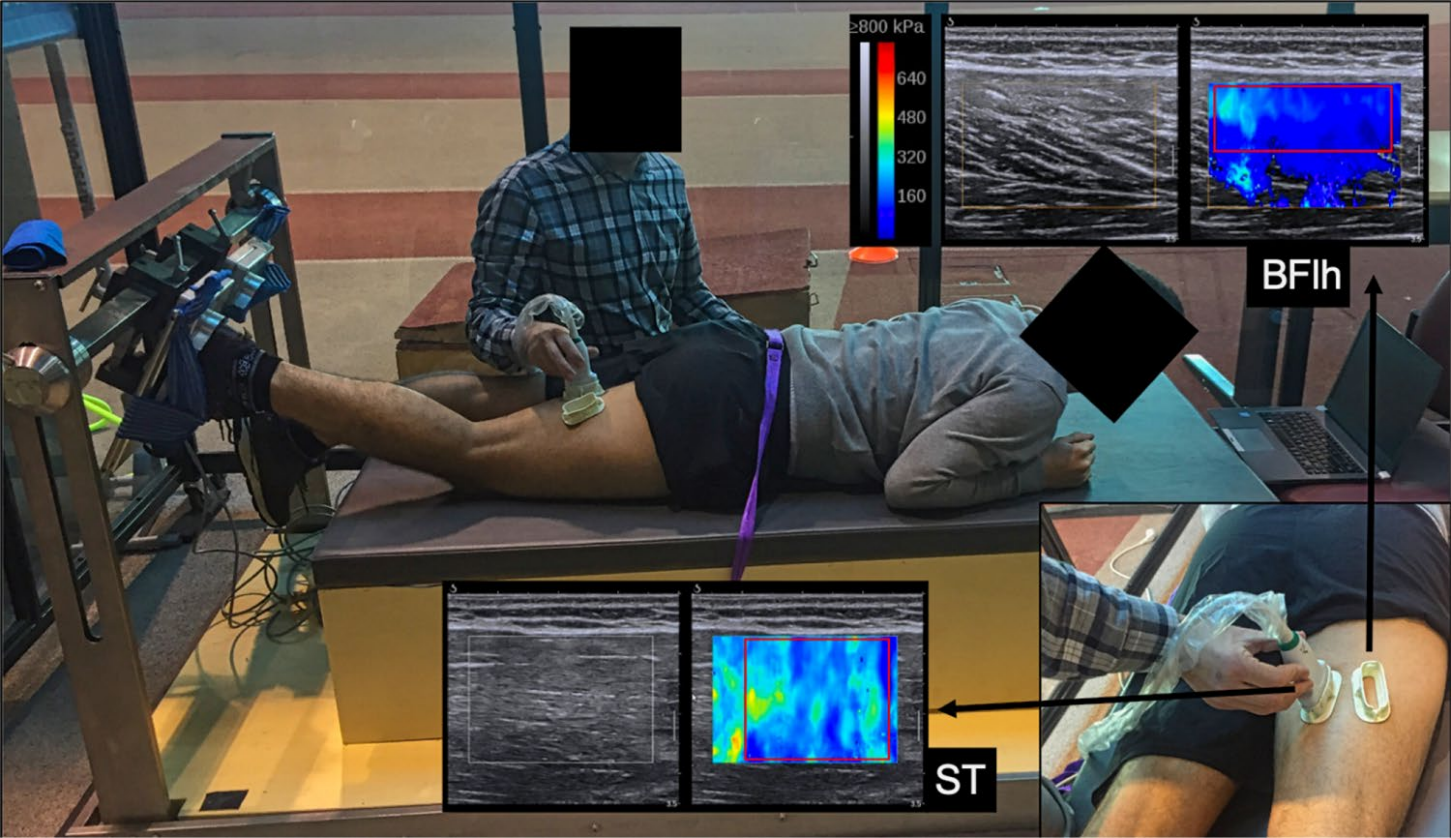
Experimental setup used to assess the localized active stiffness (i.e. shear modulus) of biceps femoris long head (BFlh) and semitendinosus (ST) during a knee flexion isometric contraction at 20% of maximal voluntary isometric contraction, with visual feedback of knee torque production during the assessments. The b-mode (left) and shear wave elastography mode (right) sonograms from one representative study individual are also shown. In the elastograms, the area of the region of interest where the analysis was performed is drawn in red. This image was performed for setup demonstration; thus, to ensure image clarity, the ultrasound scanners or the second examiner are not shown in the picture. Elastograms images were obtained from the Aixplorer's software, v10. URL: https://www.supersonicimagine.com/Aixplorer-R/Aixplorer.
